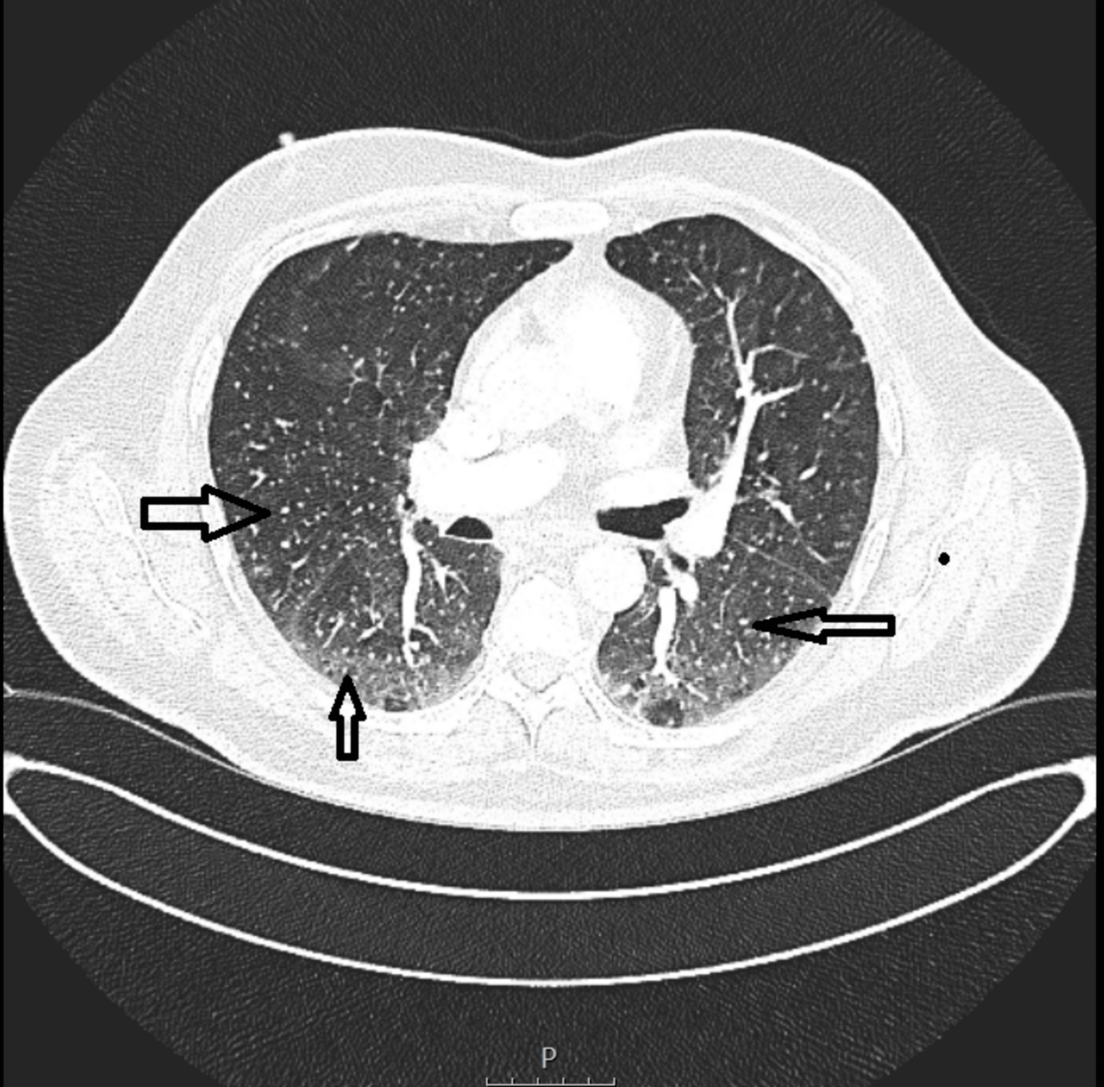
CTPA: Diffuse, uniform miliary nodules measuring 1-3 mm throughout both lungs and patchy bilateral ground-glass opacities (arrows) CTPA: computed tomography pulmonary angiography
